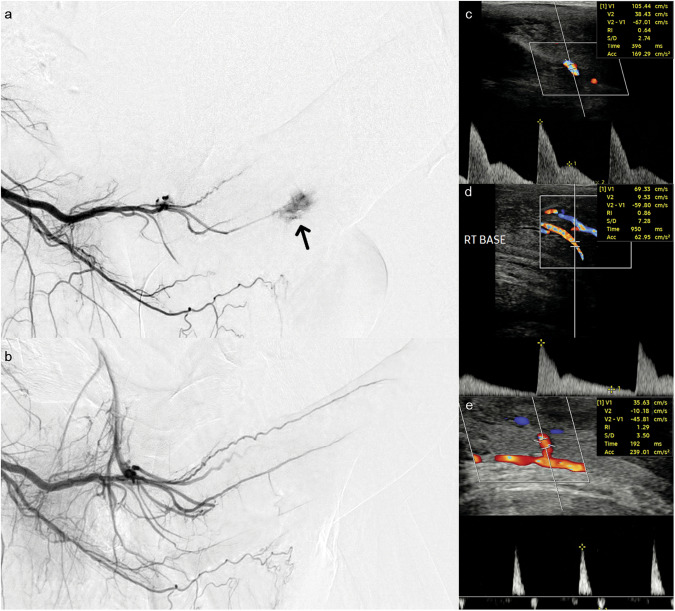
Selected angiographic and ultrasound images from a case of non-ischemic priapism. a Angiogram from the distal internal pudendal artery showing AVF (black arrow) arising from a the right cavernosal artery. b Post embolization angiogram showing resolution of the AVF and normal filling of the penile artery and branches. c Initial diagnostic CDUS trace showing a classical high flow low resistance waveform of NiP (PSV 105 cm/s, EDV 38 cm/s, RI 0.64). d Follow up CDUS at 1 week. The patient had a flaccid penis. The waveform is abnormal with the persistent forward flow in diastole (but much improved compared to the initial diagnostic scan (PSV 69 cm/s, EDV 9.5 cm/s, RI 0.86). e Subsequent CDUS at 3 months a low flow, high resistance waveform (PSV 39 cm/s, EDV -10 cm/s, RI 1.29). AVF arteriovenous fistula, NiP non-ischemic priapism, CDUS colour doppler ultrasound, PSV peak systolic velocity, EDV end diastolic velocity, RI resistive index.
